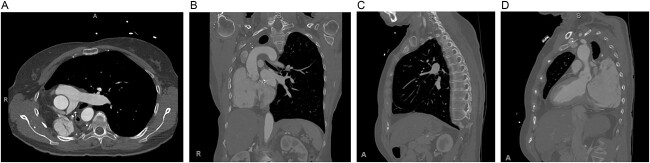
Preoperative computed tomography of the chest. Axial (A), coronal (B) and sagittal (C, D) views demonstrating displacement of the heart and left lung into the right hemithorax.
